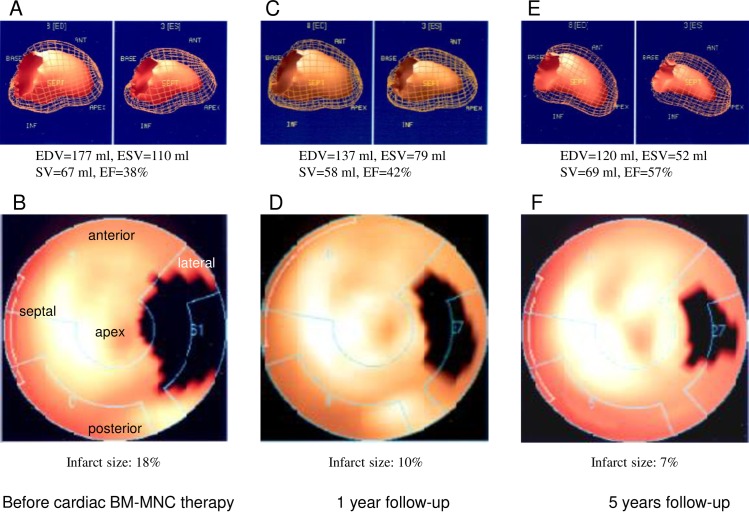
Gated ^99^m-Sestamibi myocardial scintigraphy of a patient with cardiac bone marrow mononuclear cell (BM-MNC) treatment. (A) and (B) Before combined cardiac delivery of autologous BM-MNC treatment; 3-dimensional calculation of LV volume (A) and polar map of infarct size (B). (C) and (D) At the 1-year follow-up, 3-dimensional calculation of LV volume (C) and polar map for infarct size (D). (E) and (F) At the 5-year follow-up, 3-dimensional calculation of LV volume (E) and polar map for infarct size (F).
